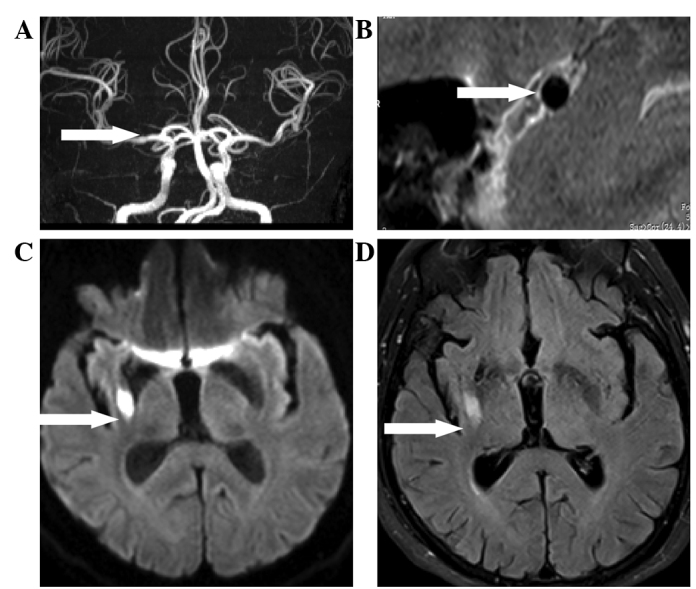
A 72-year-old male presented with left hemiparesis and dysarthria. (A) Right MCA wall MRA indicates mild-grade MCA stenosis. (B) T2-weighted HRMRI cross-sectional image of the MCA (M1 segment) reveals a normal circular lumen with no wall thickening. (C) A single small infarction of a penetrating artery is shown using DWI. (D) T2 fluid-attenuated inversion-recovery imaging indicates an infarction lesion located at the same position, corresponding to the territory of a penetrating artery of the right MCA. The arrows represent the position of the lesion. MCA, middle cerebral artery; HRMRI, high-resolution magnetic resonance imaging; DWI, diffusion-weighted imaging.
